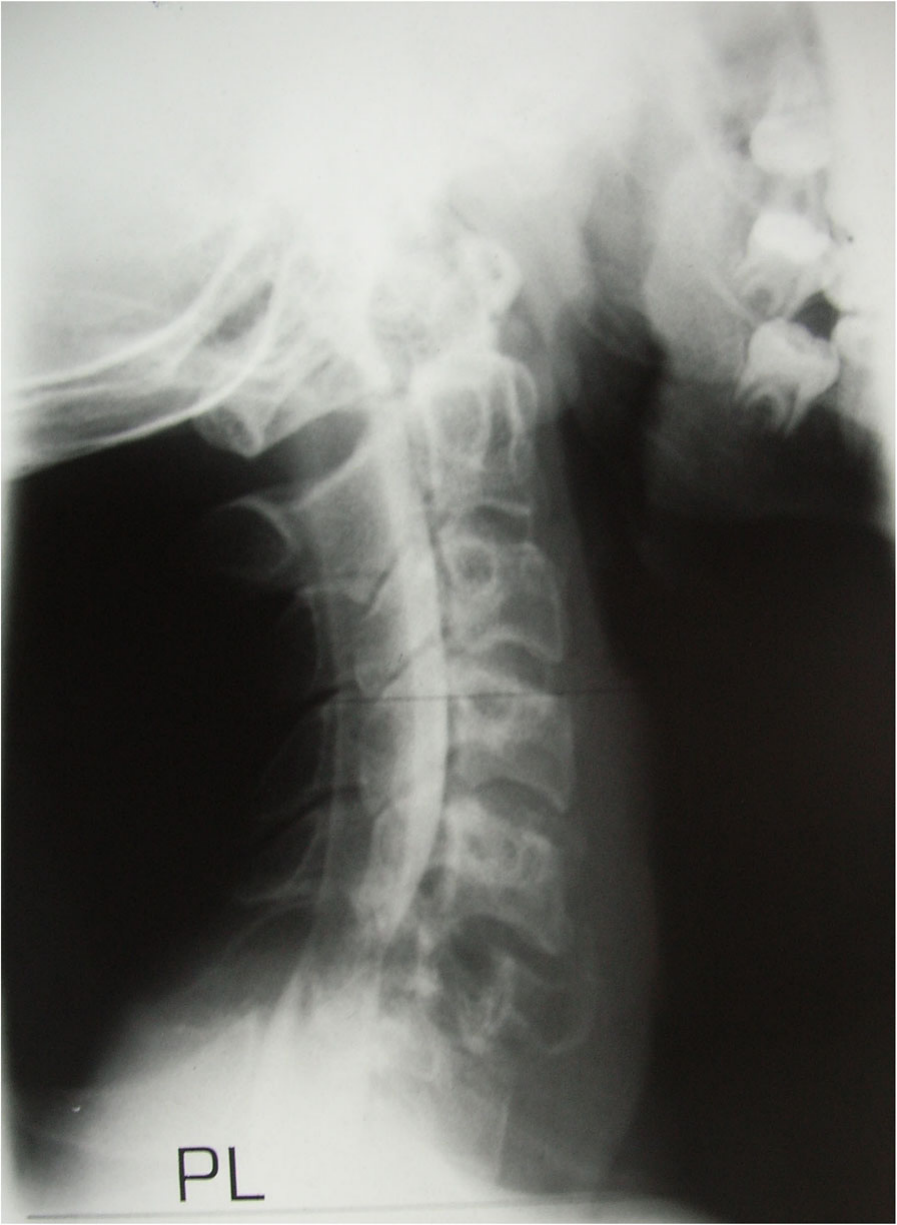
Lateral radiograph of the cervical spine of the young athlete showing the osteolytic area of C6
